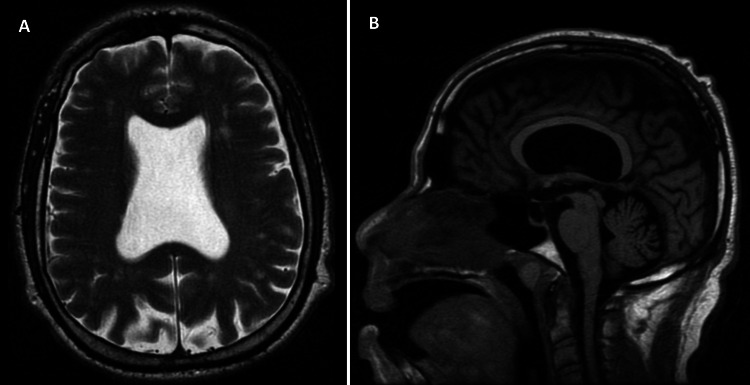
Magnetic resonance imaging of brain T2-weighted axial section (A) and T1- weighted sagittal section (B) show the absence of septum pellucidum in the lateral ventricle.
